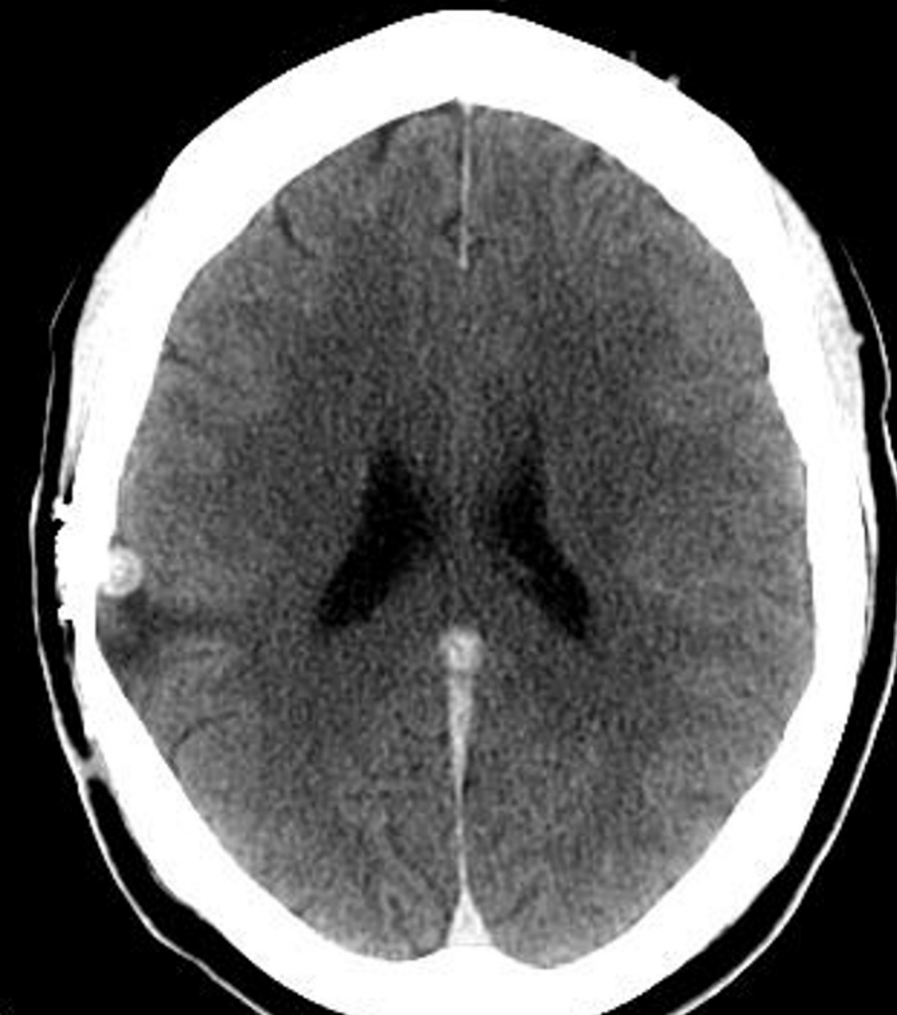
Computed tomography (CT) scan of head Computed tomography (CT) scan of head without contrast depicting hyperdensity deeper to the prior craniotomy site.
